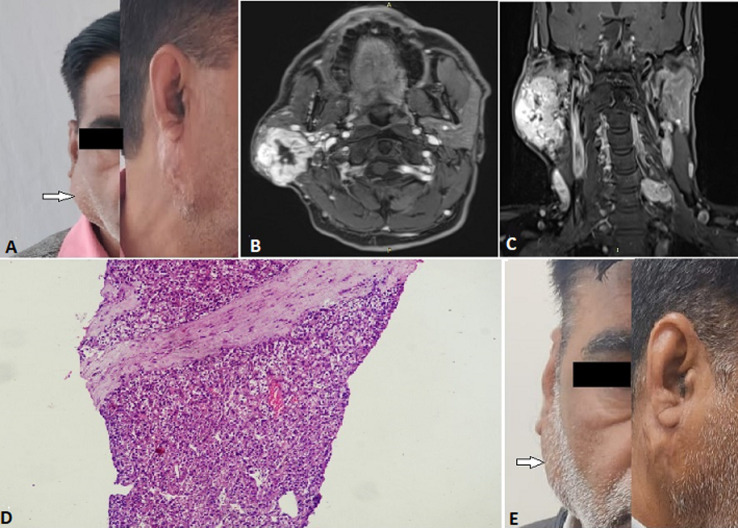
A) right parotid swelling (pre-treatment) (arrow); B) MRI inT1 showing parotid involvement; C) MRI in T2 showing the tumor involvement (coronal view); D) low power magnification (x100): fibro collagenous tissue showing infiltration by a solid tumor arranged in sheets and nests; E) right parotid swelling (post-treatment) (arrow)
